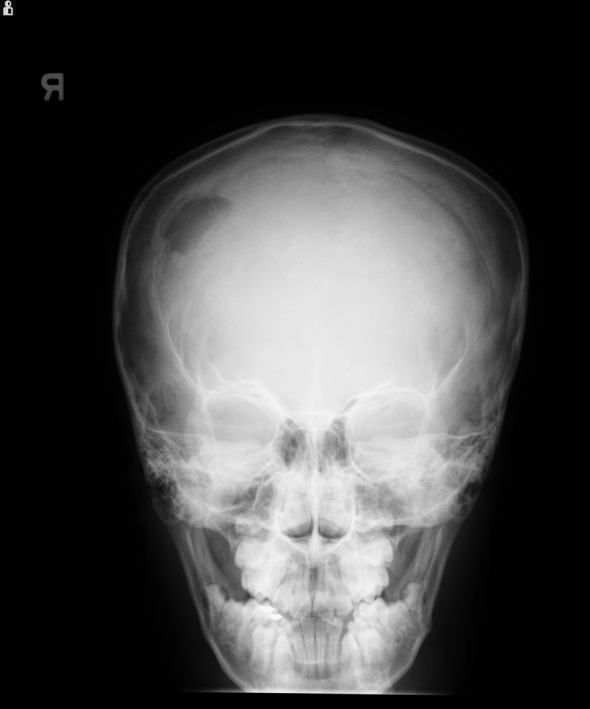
X-ray of the skull showing lytic lesion in the right parietal region.
